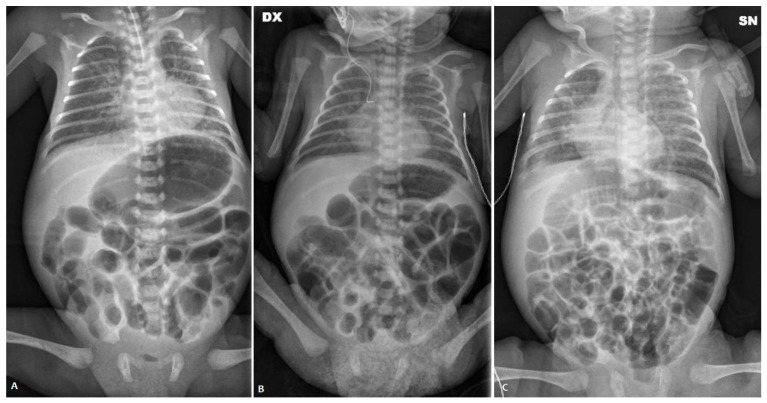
Abdominal X-ray of patient P3 on the 3rd day of life (A); abdominal X-ray before the NAC treatment (B) and after the NAC treatment (C).
